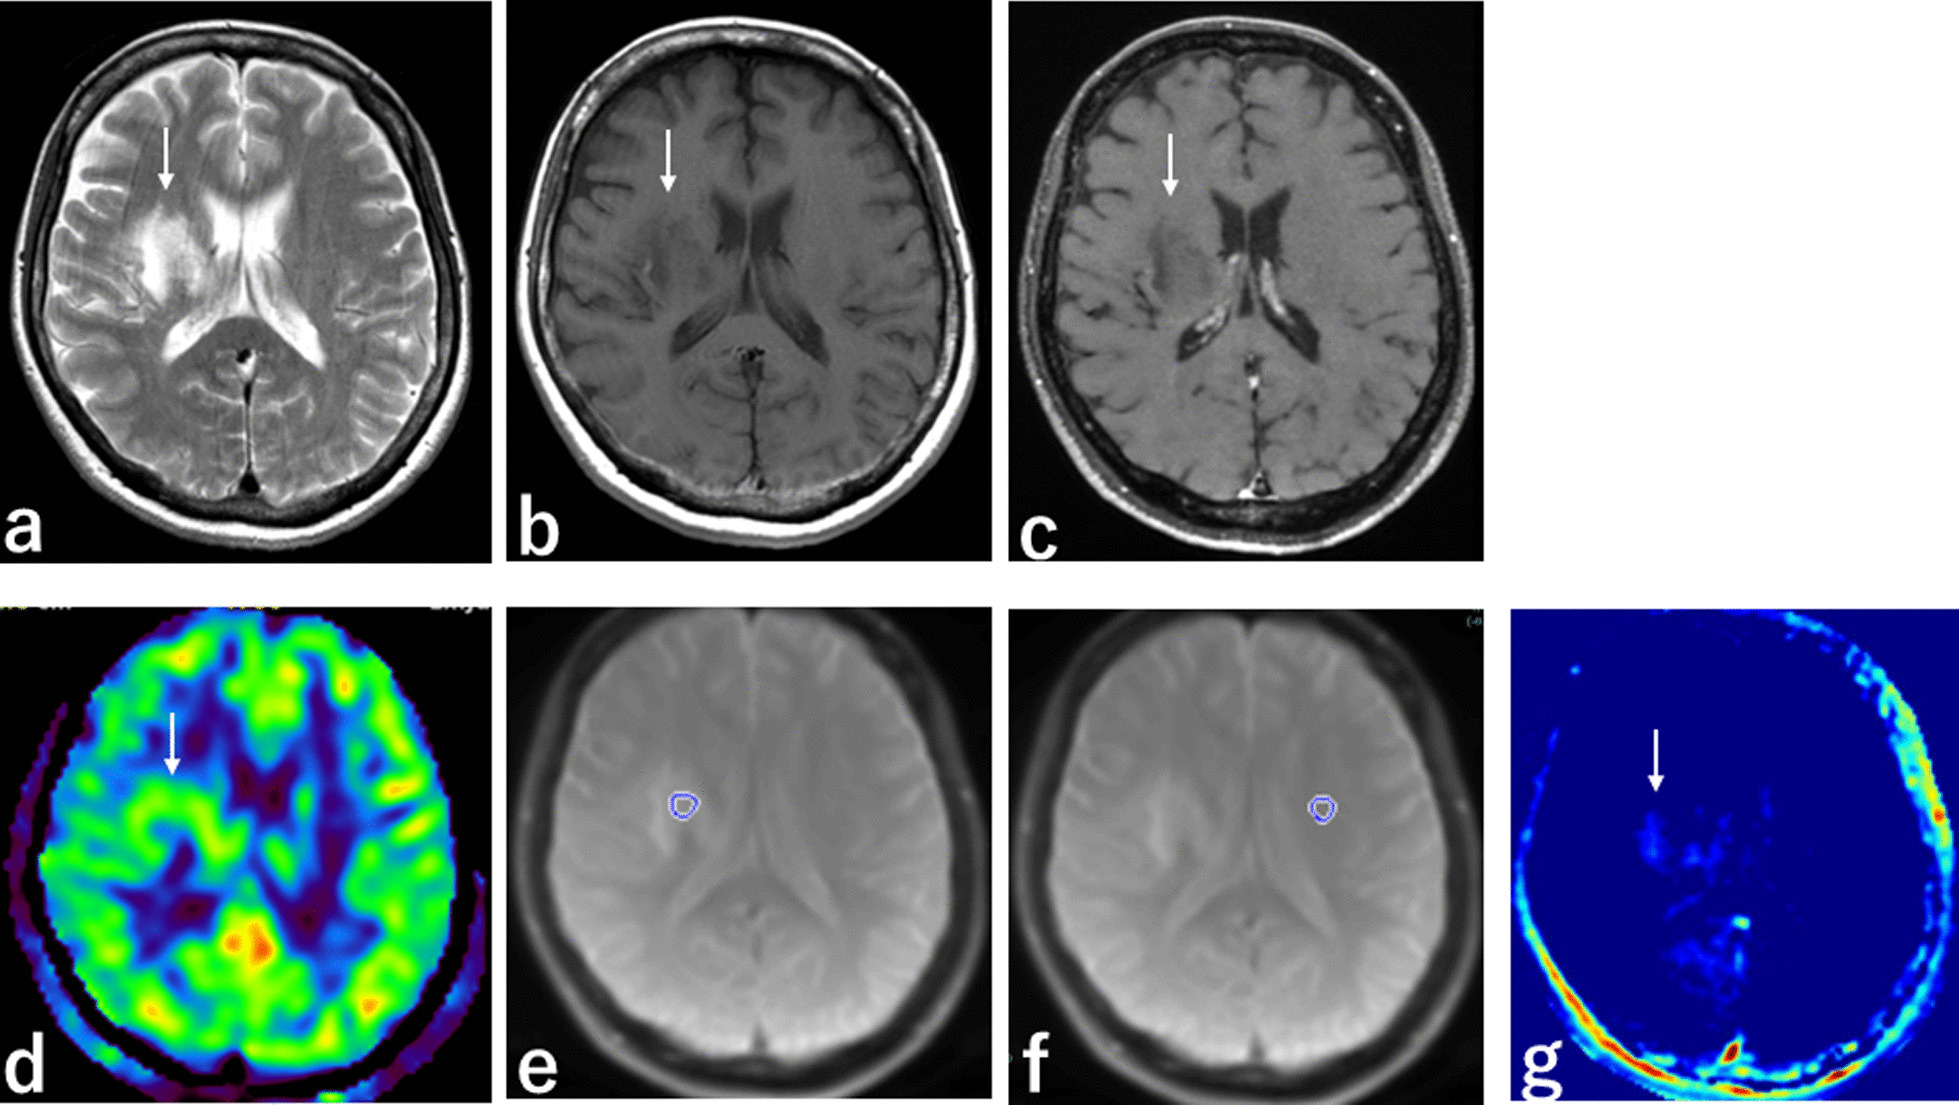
MRI from a 53-year-old woman with PML. a T2-weighted sequence, axial section, shows poor definition and high SI in PML in the white matter of the right frontal lobe (white arrow). b T1-weighted sequence, axial section, shows poor definition and low SI in PML in the white matter of the right frontal lobe (white arrow). c 3D contrast-enhanced T1-weighted sequence, axial section, shows no enhancement in PML. d 3D ASL sequence, axial section, shows isointensity in PML. e APT scout image, axial section, shows the ROI placed in PML. f APT scout image, axial section, shows the ROI placed in normal-appearing tissue contralateral to lesion. g APT imaging shows low SI in PML (white arrow). *PML* progressive multifocal leukoencephalopathy, *SI* signal intensity, *ASL* arterial spin labelling, *APT* amide proton transfer
